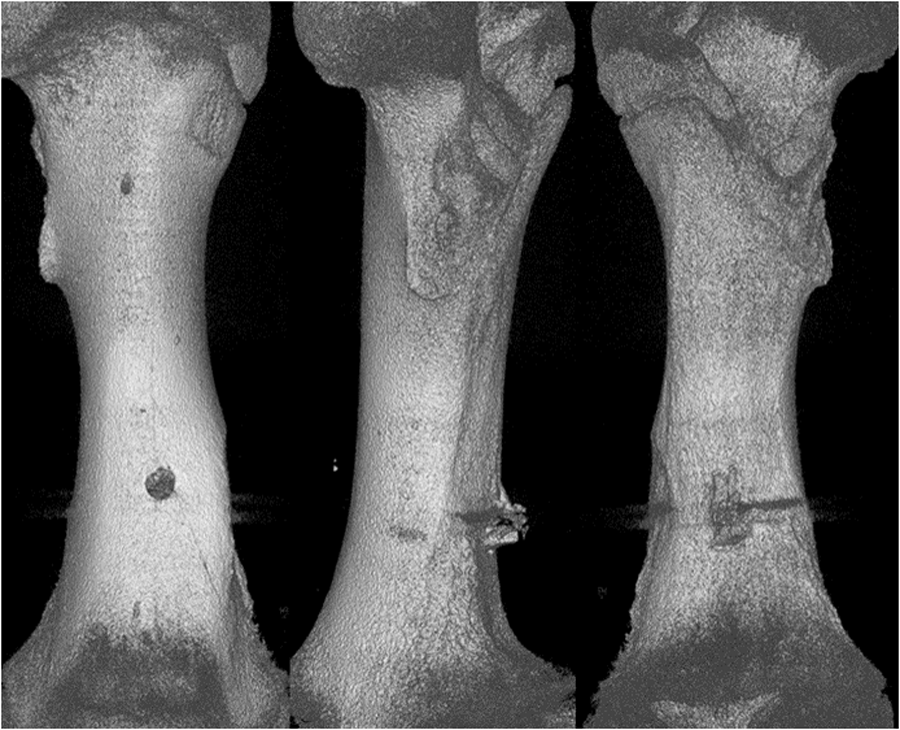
H&N Excite Apollo cal. 5.5 mm. 3D CT gunshot damage reconstruction. From the left: anterior, lateral and posterior shaft surfaces.
